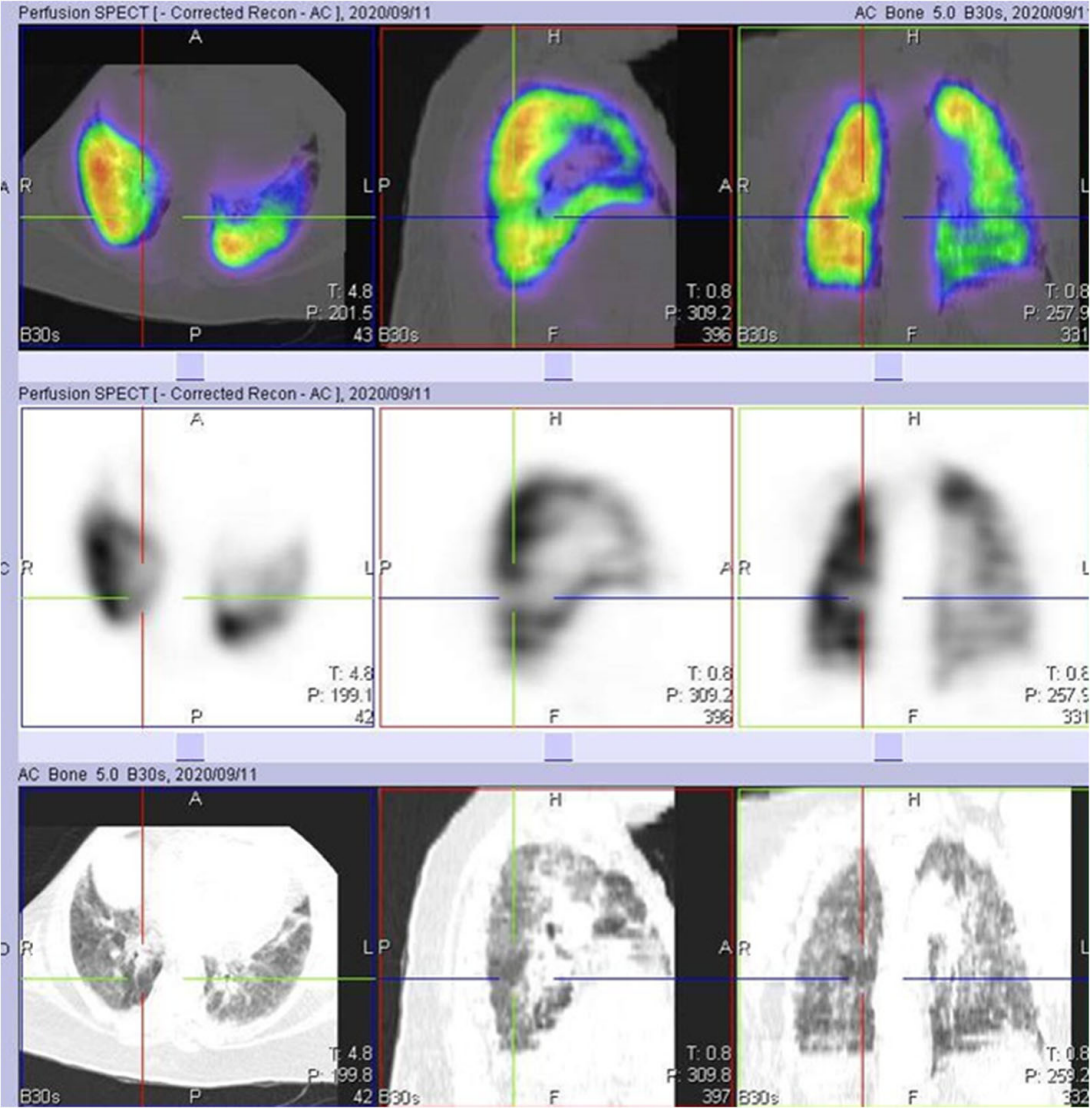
Perfusion only SPECT/CT image of a 32-year-old female, performed 18 days after testing positive for COVID-19 infection, showing a perfusion defect in the right lung (crosshair) that appears to be associated with shunting
